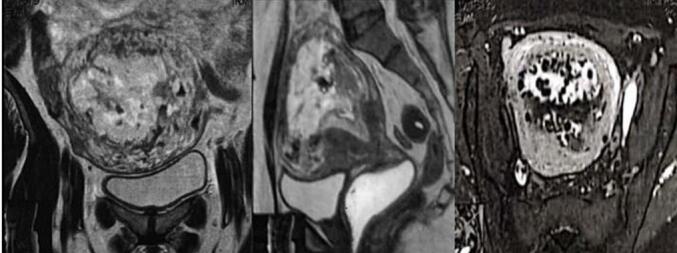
Magnetic resonance imaging view showing a poorly defined intracavitary mass of heterogeneous signal associated with cervicoisthmic endometrial thickening.
